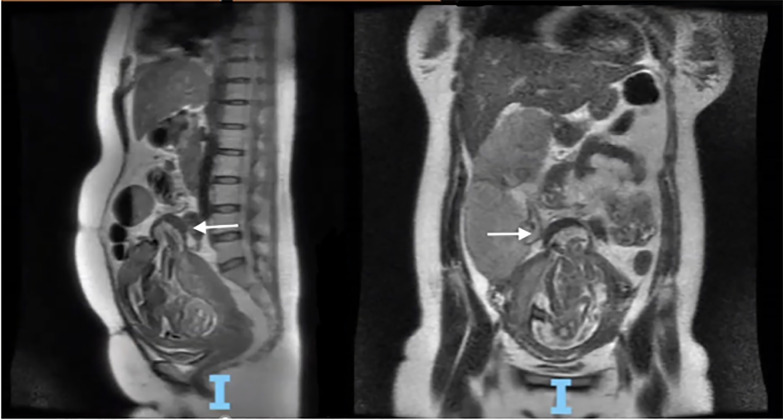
Magnetic resonance imaging with white arrow demonstrating a sac-like structure measuring 4.4 centimeters in the fundal portion of the uterus, containing a portion of gestational sac and pregnancy contents with intact serosa seen in the sagittal and coronal views, respectively.
